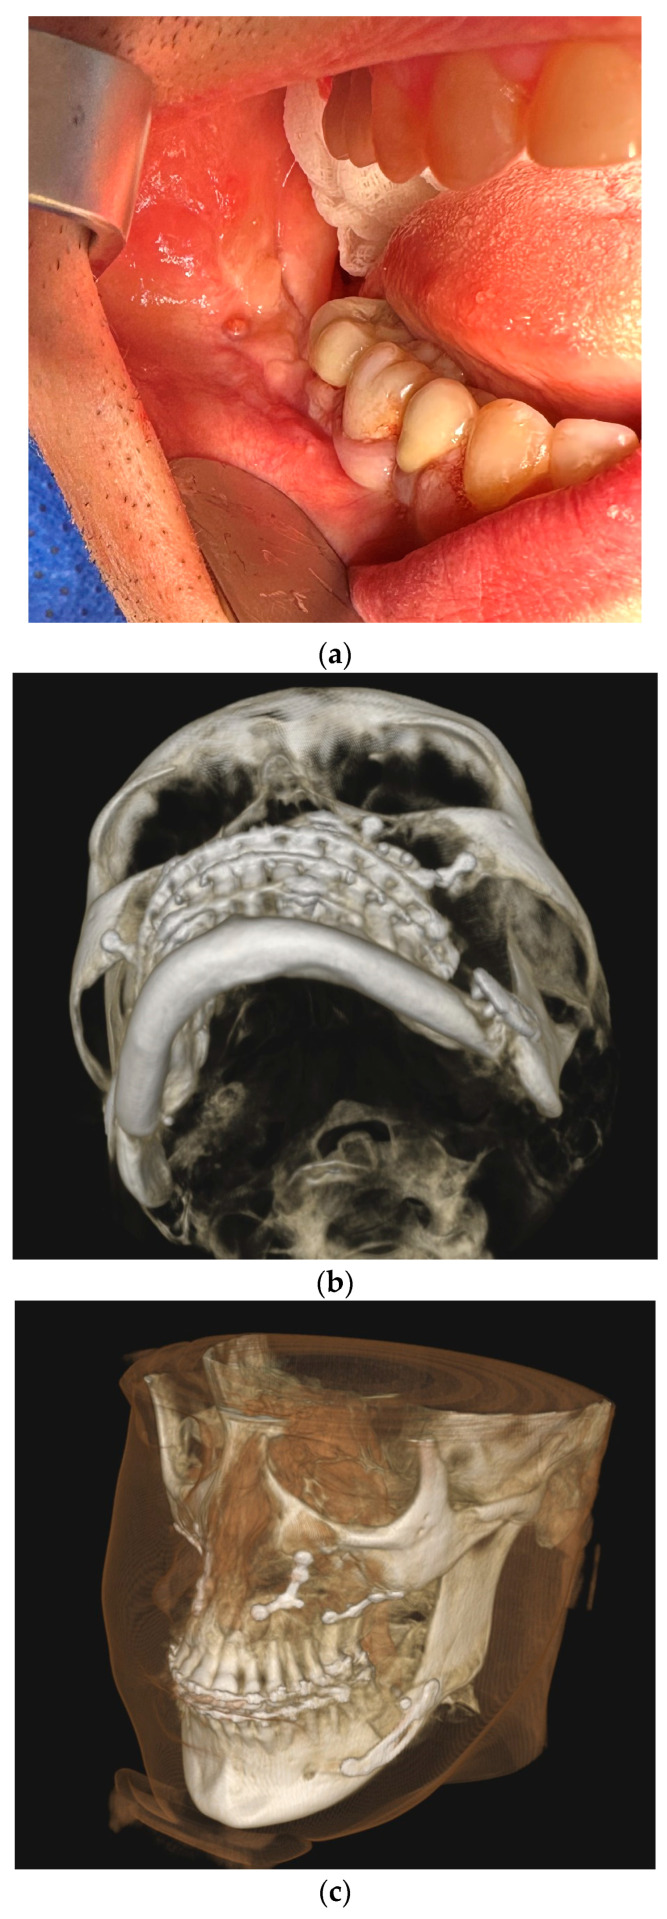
Image of inflammation/infection: (a) intraoral photo with fistula in the fixation area; (b) CBCT mandibular screws not fixed due to inflammation; (c) CBCT osteonecrosis in the bicortical screw area.
